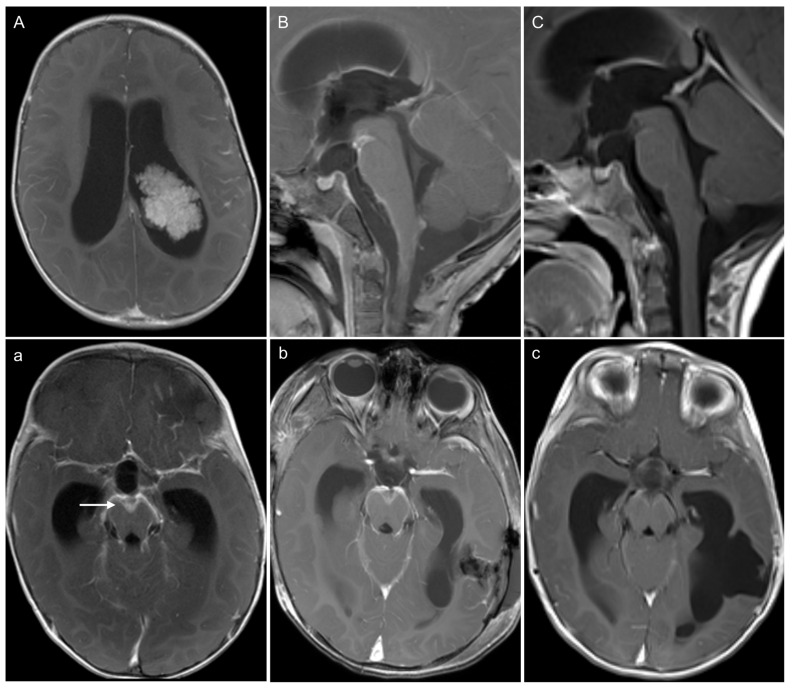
A 1-year-old male CPP patient with pseudo leptomeningeal dissemination at diagnosis. Contrast-enhanced T1-wheigted images show the course from presurgery to pre-chemotherapy in sagittal (A–C) and transverse planes (a–c). (A,a) Contrast enhanced T1-wheighted image shows the primary tumor and the diffuse leptomeningeal contrast enhancement at the skull base, especially in the interpeduncular cistern as demonstrated in (arrow in a). (B,b) The diffuse contrast enhancement was still detectable immediately after surgery. (C,c) However, the contrast enhancement had regressed in an MRI performed 4 weeks later before the start of chemotherapy.
